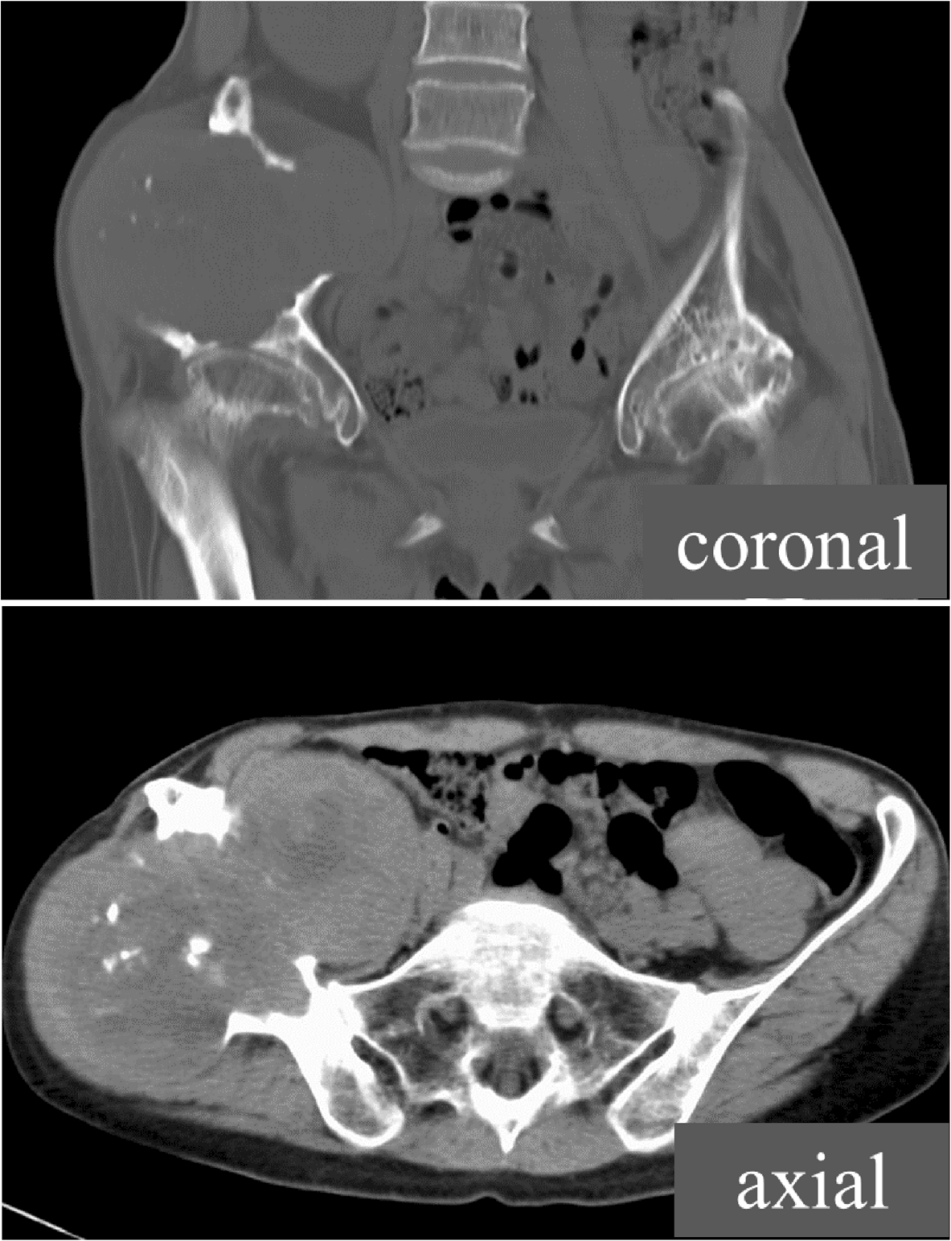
Computed tomography of the ilium. Initial computed tomography demonstrated a heterogeneous mass around the ilium and an area of destroyed bone
